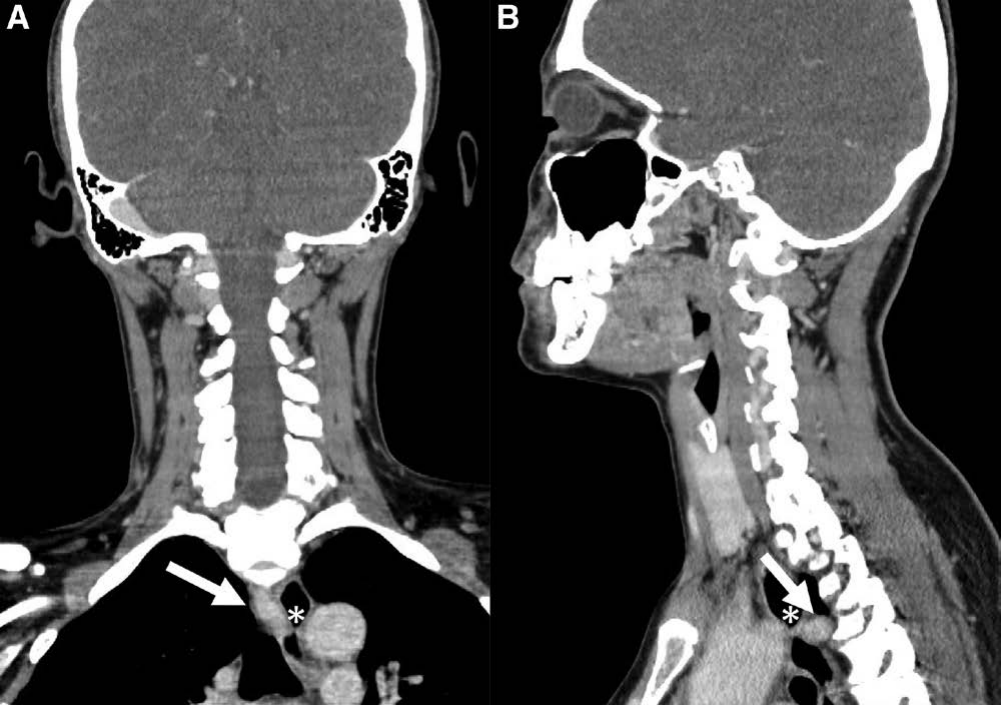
(A) Coronal and (B) sagittal contrast-enhanced computed tomography images show esophageal compression (asterisks) caused by the aberrant right subclavian artery (arrows).
